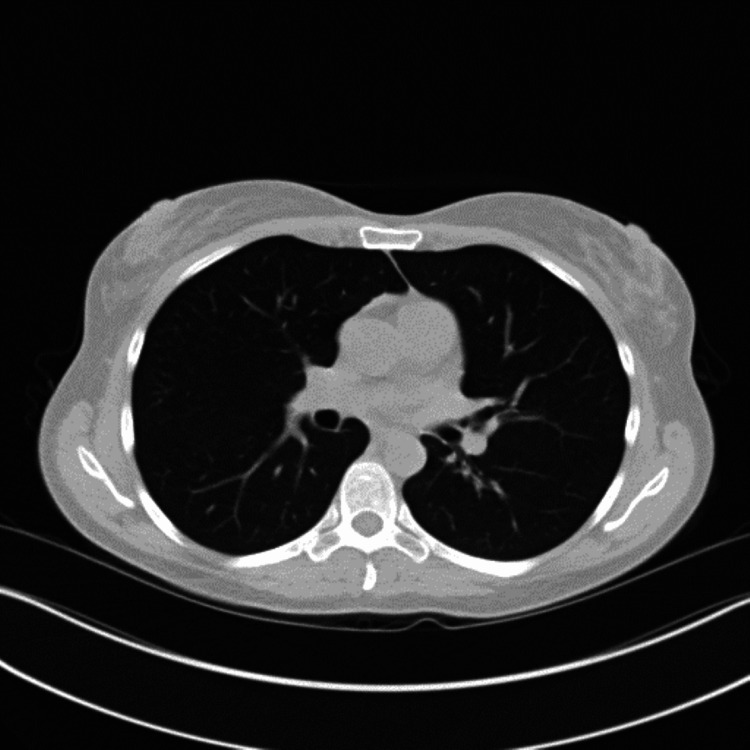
CT chest showing interval resolution of previously seen ground-glass opacities
